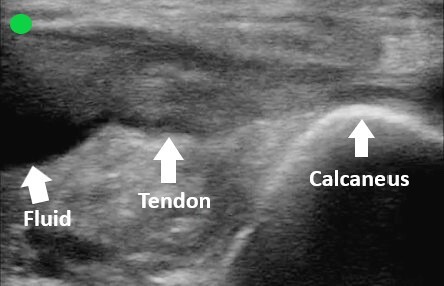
Sagittal view of the distal Achilles tendon. Point-of-care ultrasound image obtained with a high-frequency linear probe with the probe marker directed cephalad. An anechoic fluid collection is present just proximal to where the Achilles tendon attaches to the calcaneus.
